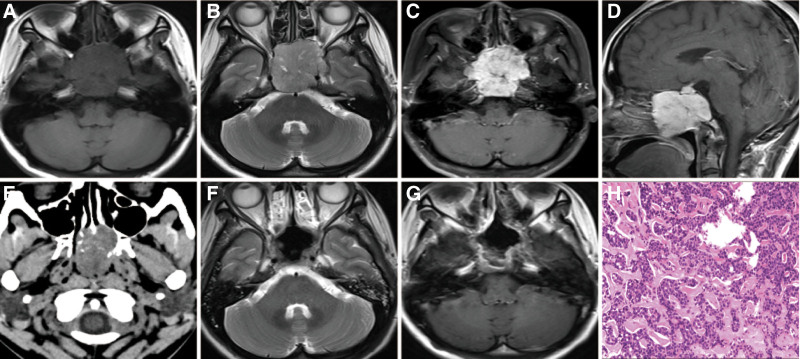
Case 11. Magnetic resonance images showing isointense T2 (A) isointense T1 (B) in the slope and sphenoid sinus and with the heterogeneous enhance (C and D). Bone destruction and the mixed-density lesion were observed on computed tomography (E). Magnetic resonance images (F and G) revealing gross remove the tumor. Pathological examination (H) revealed the diagnosis of ACC. H & E, original magnification = 200×. ACC = adenoid cystic carcinoma.
